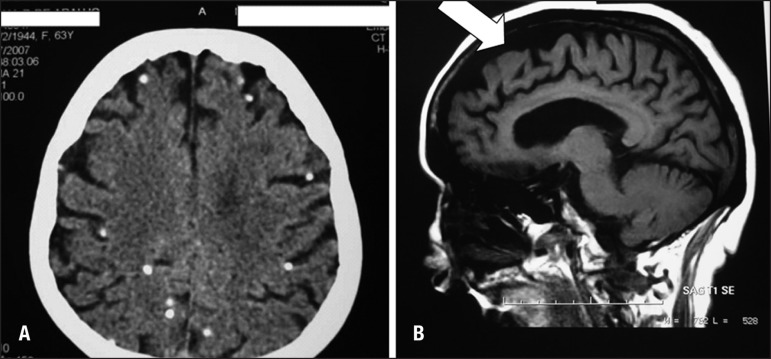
[A] Unenhanced computed tomography (CT Scan of the brain) showing
